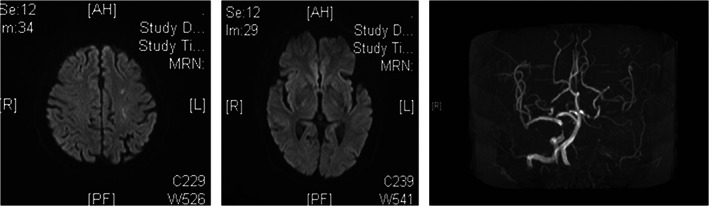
DWI images only showing a minimum high‐intensity lesions in the left frontal lobe and MRA revealed severe stenosis or occluded of the left ICA and stenosis of the right ICA.
